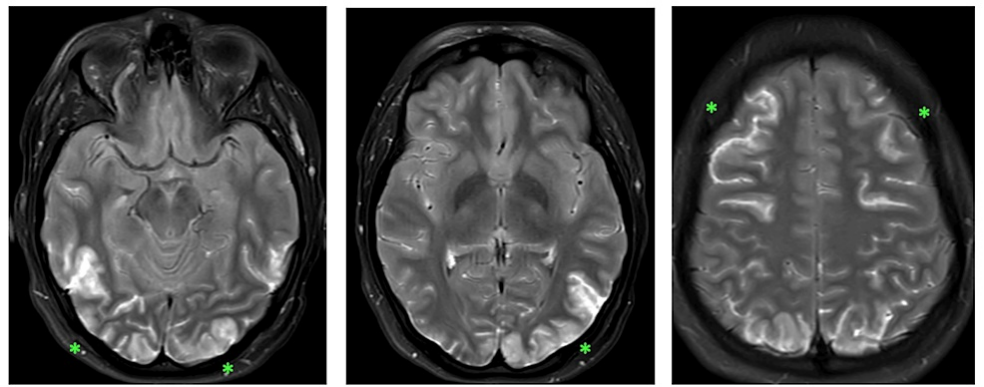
Axial T2-Weighted MRI MRI (magnetic resonance imaging). Bilateral near-symmetrical areas of increased signal abnormality in the frontal and occipital regions (*).
